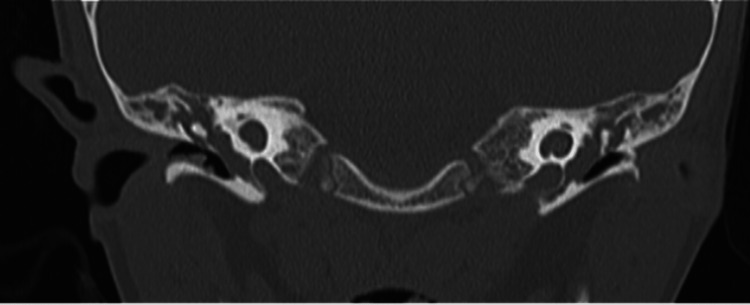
CT temporal bone coronal view showing bilateral cystic vestibule and bilateral cystic apical and middle turns of the cochlea.
